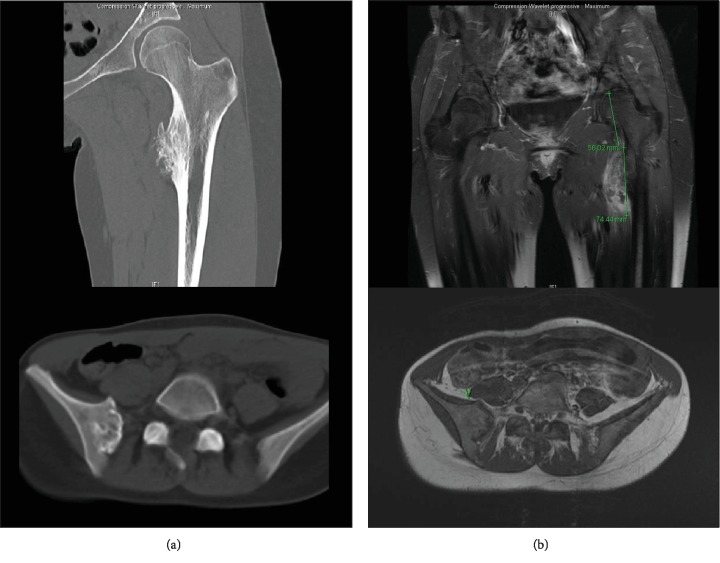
Lesions of the right sacroiliac and of the lesser trochanter: (a) aspect on CT scan and (b) aspect on MRI T1 Gado FAT SAT.
